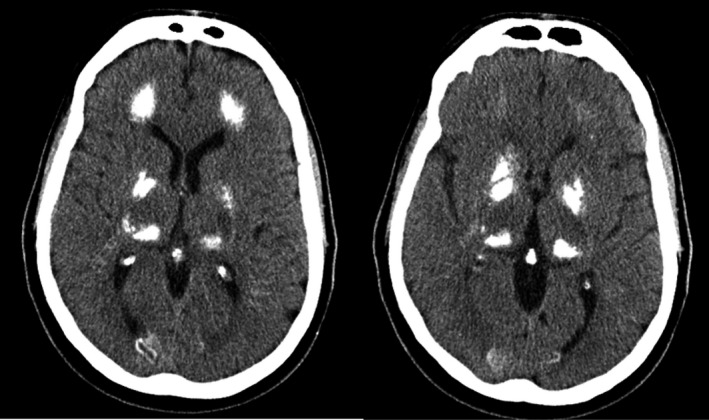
CT scan in the axial plane showing extensive bilateral calcification of basal ganglia.
